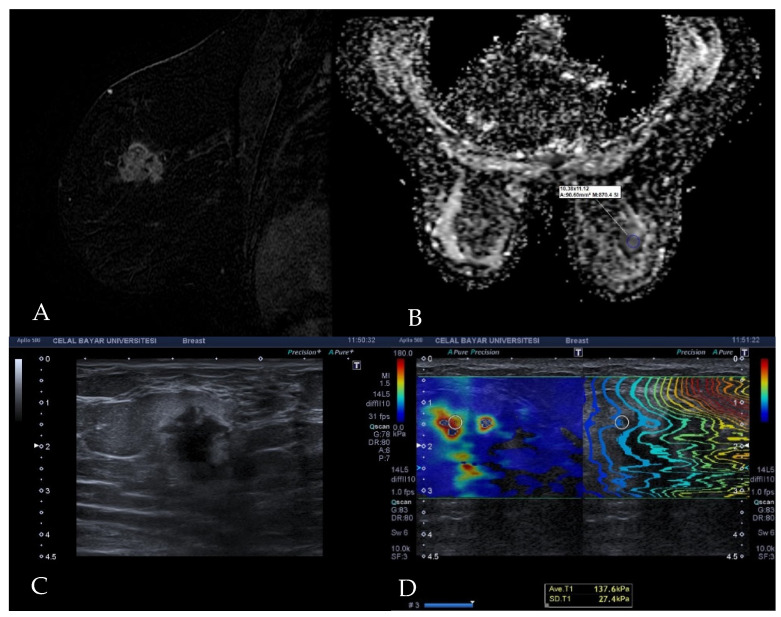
Images of invasive ductal carcinoma in a 36-year-old female patient. (A) Contrast-enhanced sagittal T1w image demonstrates a mass with irregular shape and contours and heterogeneous enhancement. (B) The lesion has a low signal on the ADC map with the value of 0.77 × 10^−3^ cm^2^/s. (C) B-mode ultrasonography shows a hypoechoic mass with angular margins. (D) Shear wave elastography reveals the mass is stiff with an elasticity value of 137.6 kPa.
